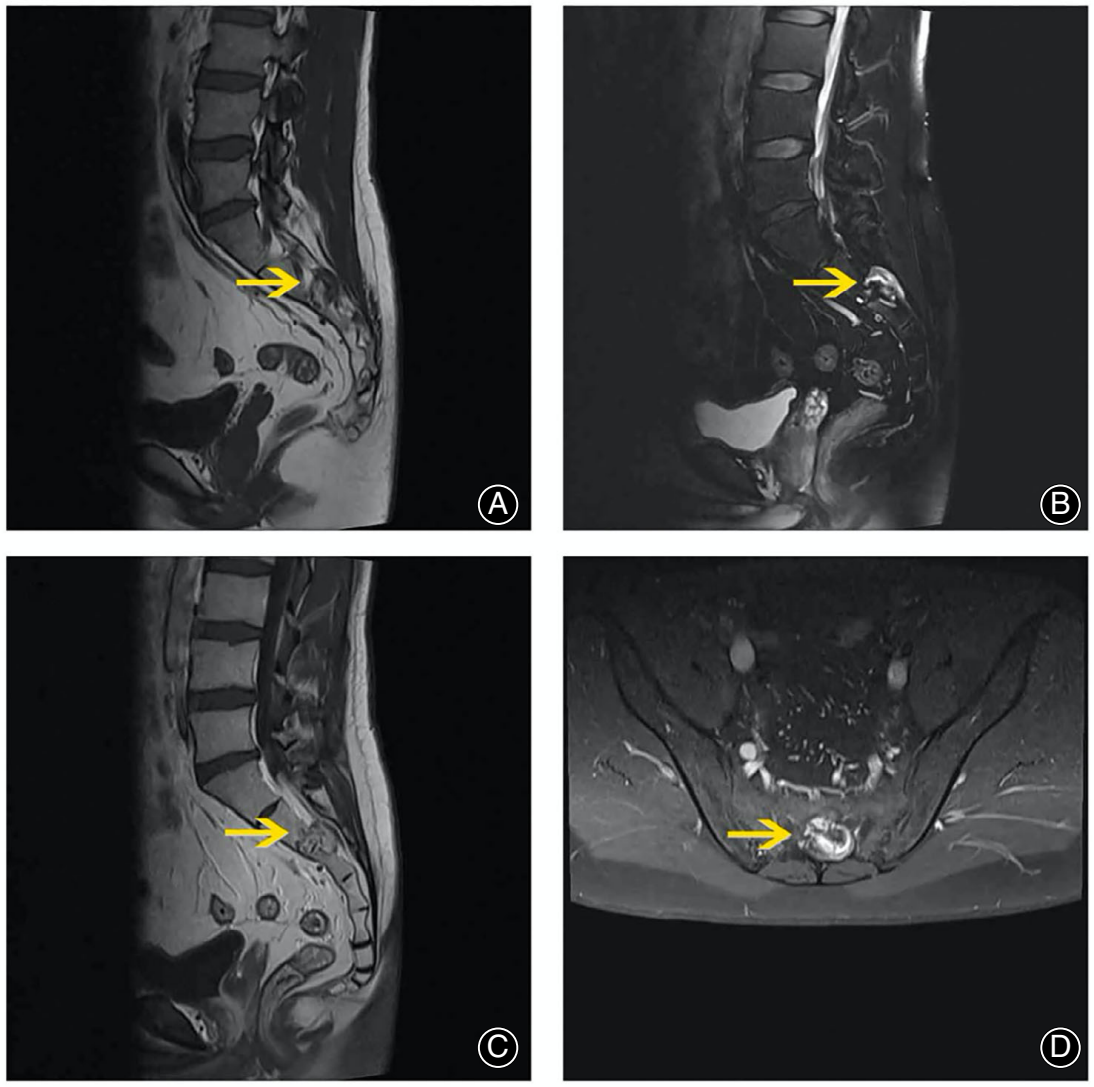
A destructive malignancy occupying spinal canal in the sacrum (arrow) was revealed in the sagittal view of lumbosacral spine MRI (A). The lesion displayed mixed signal intensity on T1‐weighted MRI (T1WI; A) and T2‐weighted MRI (T2WI; B, D). Contrast‐enhanced T1‐ weighted MRI (CET1; C) revealed a remarkable contrast enhancement
